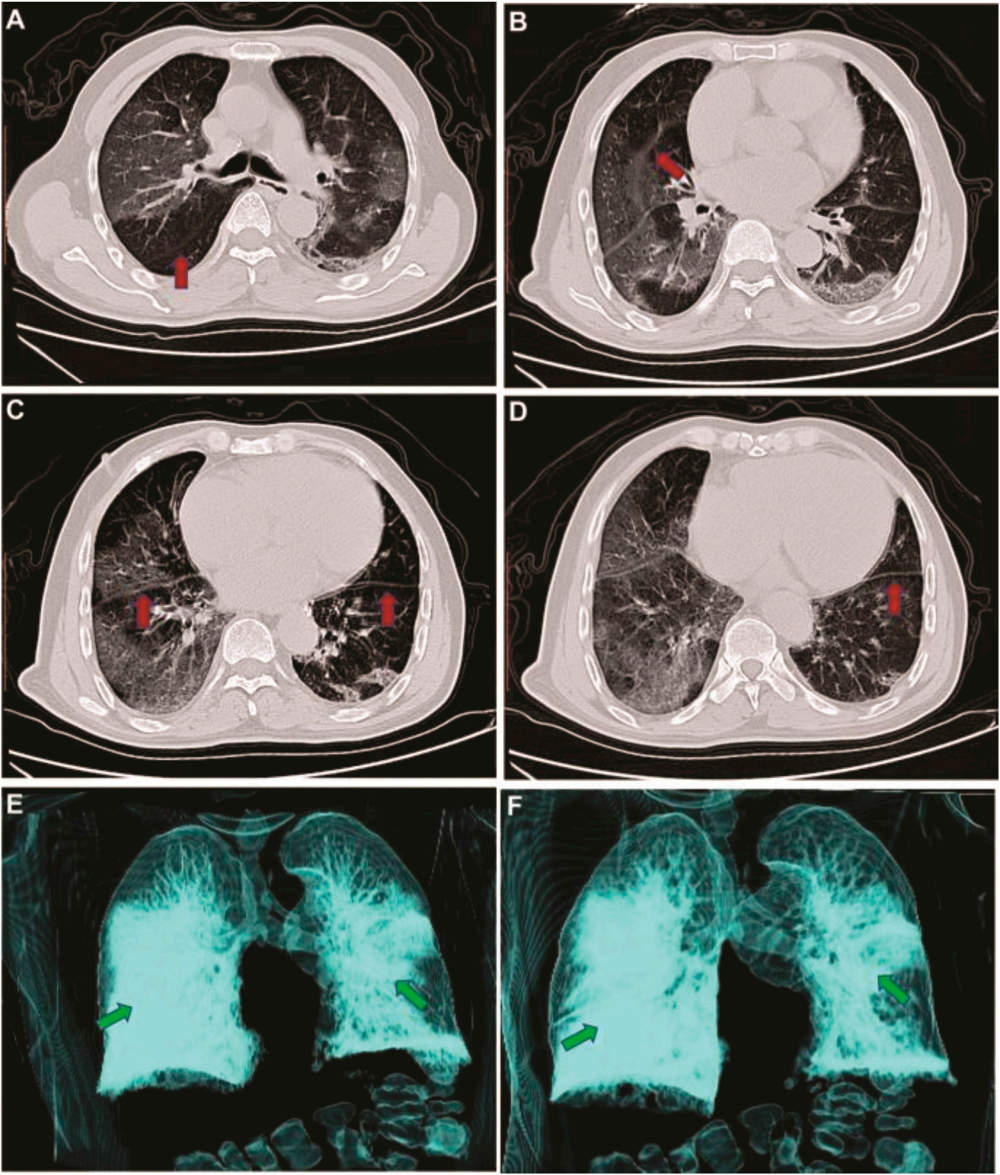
CT image changes of an infected patient with COVID-19. Panel A, B, and C indicate CT images on day 5 after symptoms onset, and D, E, and F represent on day 20 after symptoms onset. Adapted from Zhang, W. Imaging changes in severe COVID-19 pneumonia. Intensive Care Med [212].
